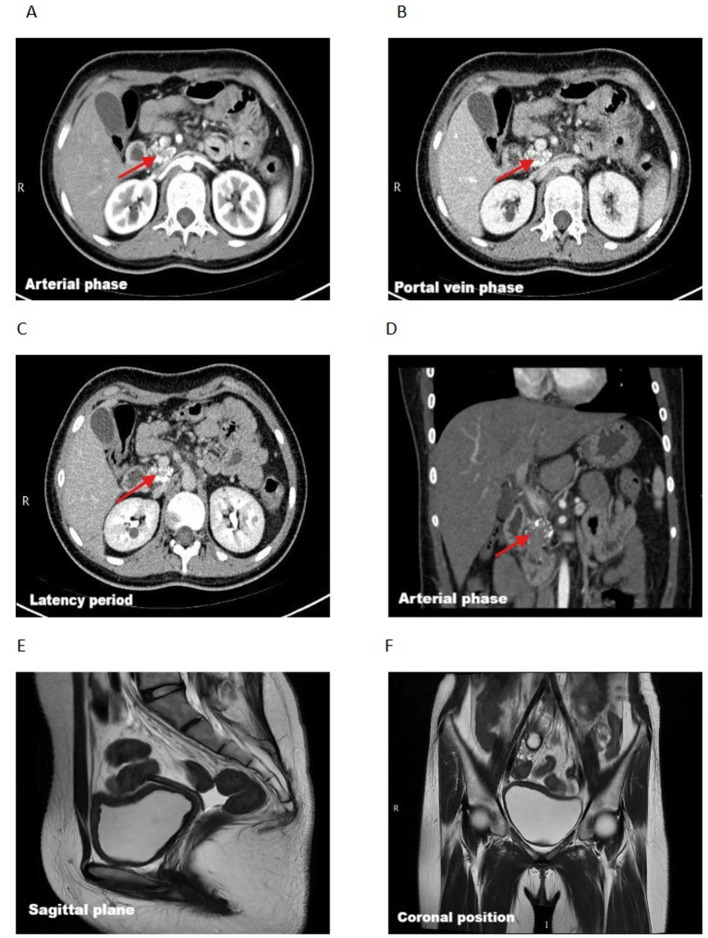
Bdominal contrast-enhanced CT and pelvic MRI findings. (A–C) Axial contrast-enhanced CT images obtained during the arterial phase (A), portal venous phase (B), and delayed phase (C) demonstrate multiple patchy calcifications in the uncinate process of the pancreatic head (red arrows). (C) In the delayed phase, bilateral kidneys show multiple rounded hypodense non-enhancing lesions, consistent with renal cysts, with the largest measuring approximately 1.4 cm in diameter. (D) Coronal CT reconstruction shows marked pancreatic atrophy with non-visualization of the pancreatic body and tail (red arrow). (E) Pelvic MRI sagittal view shows absence of the uterus with poorly defined vaginal structures. (F) Pelvic MRI coronal view demonstrates bilateral ovaries located adjacent to the psoas major muscles.
